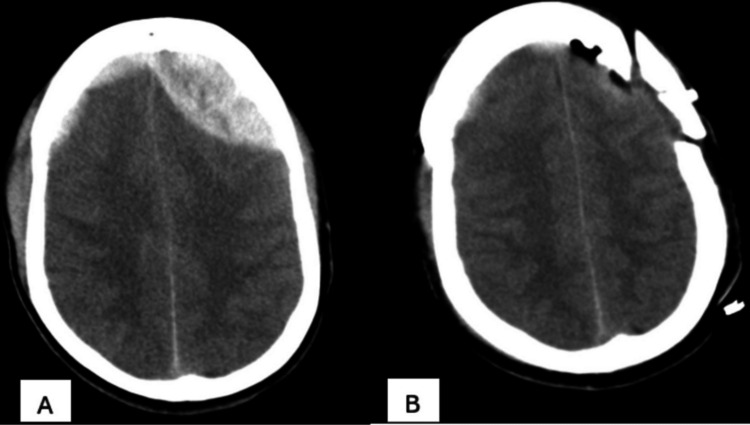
(A) Plain CT scan of brain showing bifrontal acute extradural hematoma, possibly due to shearing pressure on anterior superior sagittal sinus. (B) Craniotomy flap seen with complete evacuation of acute extradural hematoma.
